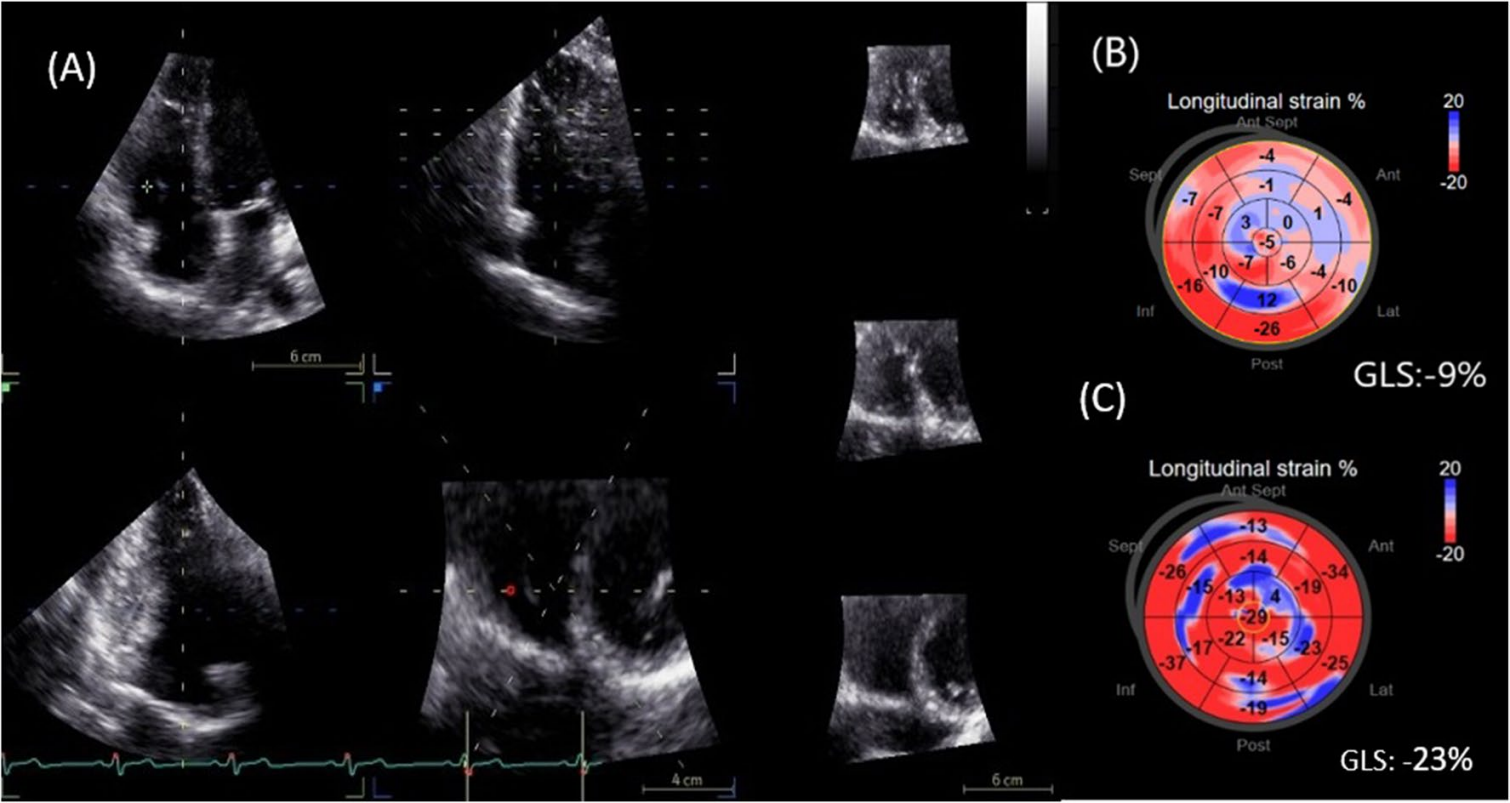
Representative RV 3D-STE analysis in two patients with OSA. (A) Automatic RV 3D-STE analysis using 4DAutoLVQ function. (B) Bull’s eyes reconstruction of 3D GLS in a patient with severe OSA ( decrease of GLS =  − 9%) respectively, in a healthy volunteer ( GLS =  − 23%). RV, right ventricle; 3D, three-dimensional; STE, speckle-tracking echocardiography; OSA, obstructive sleep apnea; GLS, global longitudinal strain.
